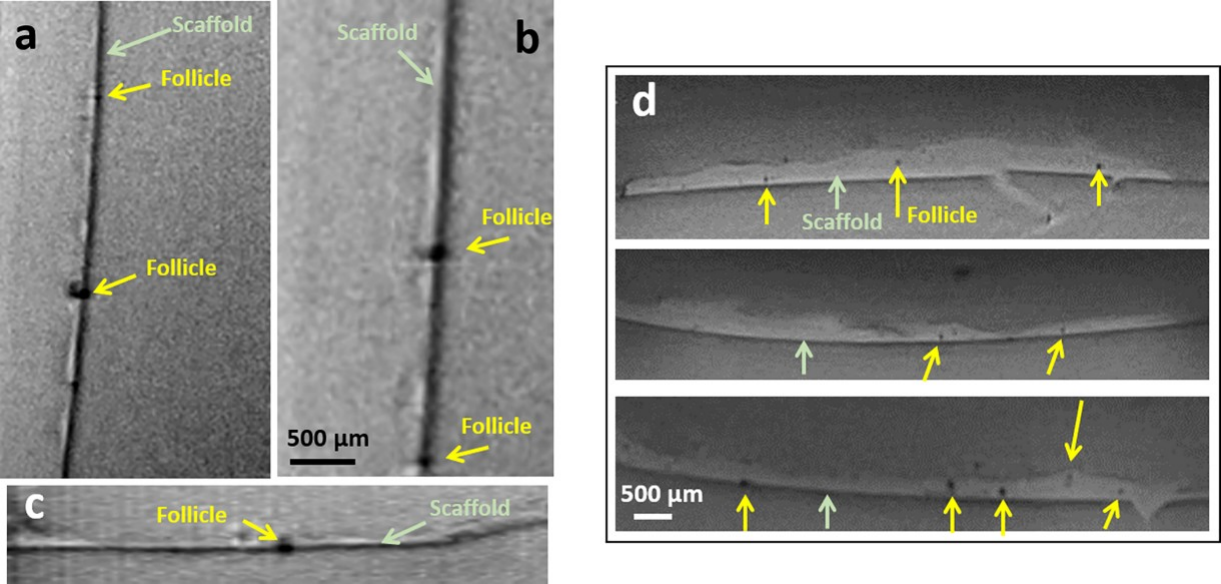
MR images of agarose embedded scaffolds with a resolution of 23x23x80μm^3^ (a,b,c) PCL scaffold and (d) PCL/gelatin scaffold, scale bar 500 μm.
